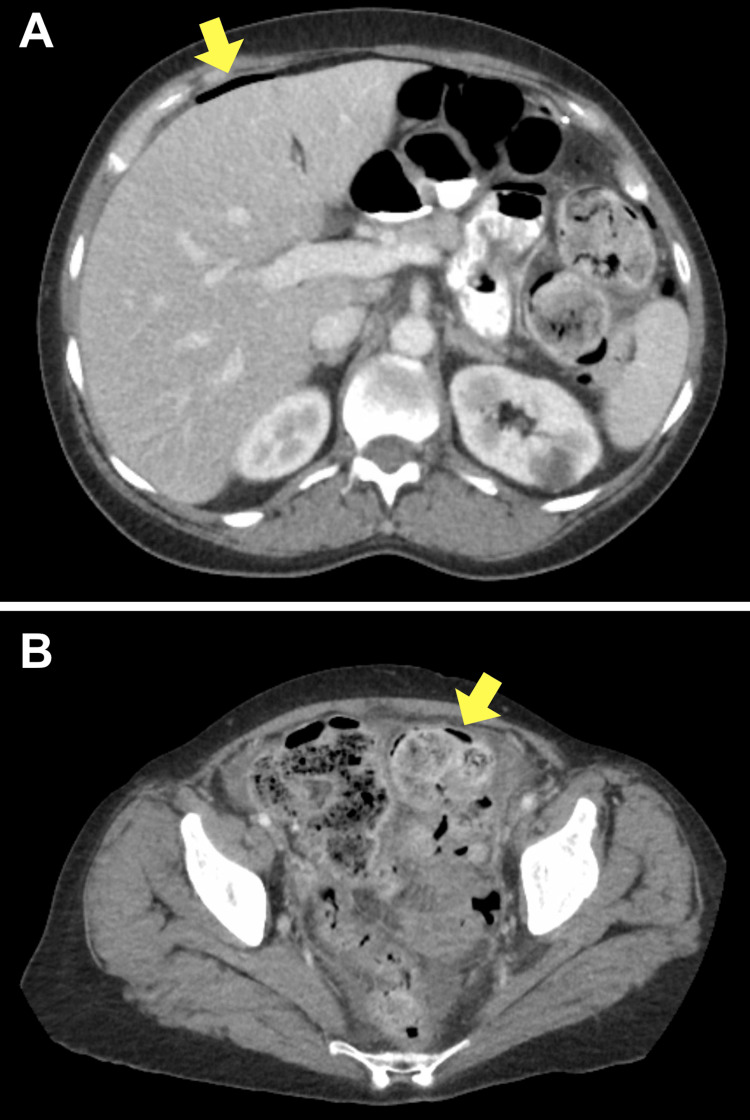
Abdominal computed tomography scan of a 50-year-old female with perforated diverticulitis (A) Axial image with abdominal free air anterior to the liver (arrow); (B) Axial image with wall thickening in the sigmoid colon and possible air in the sigmoid colon wall (arrow)
